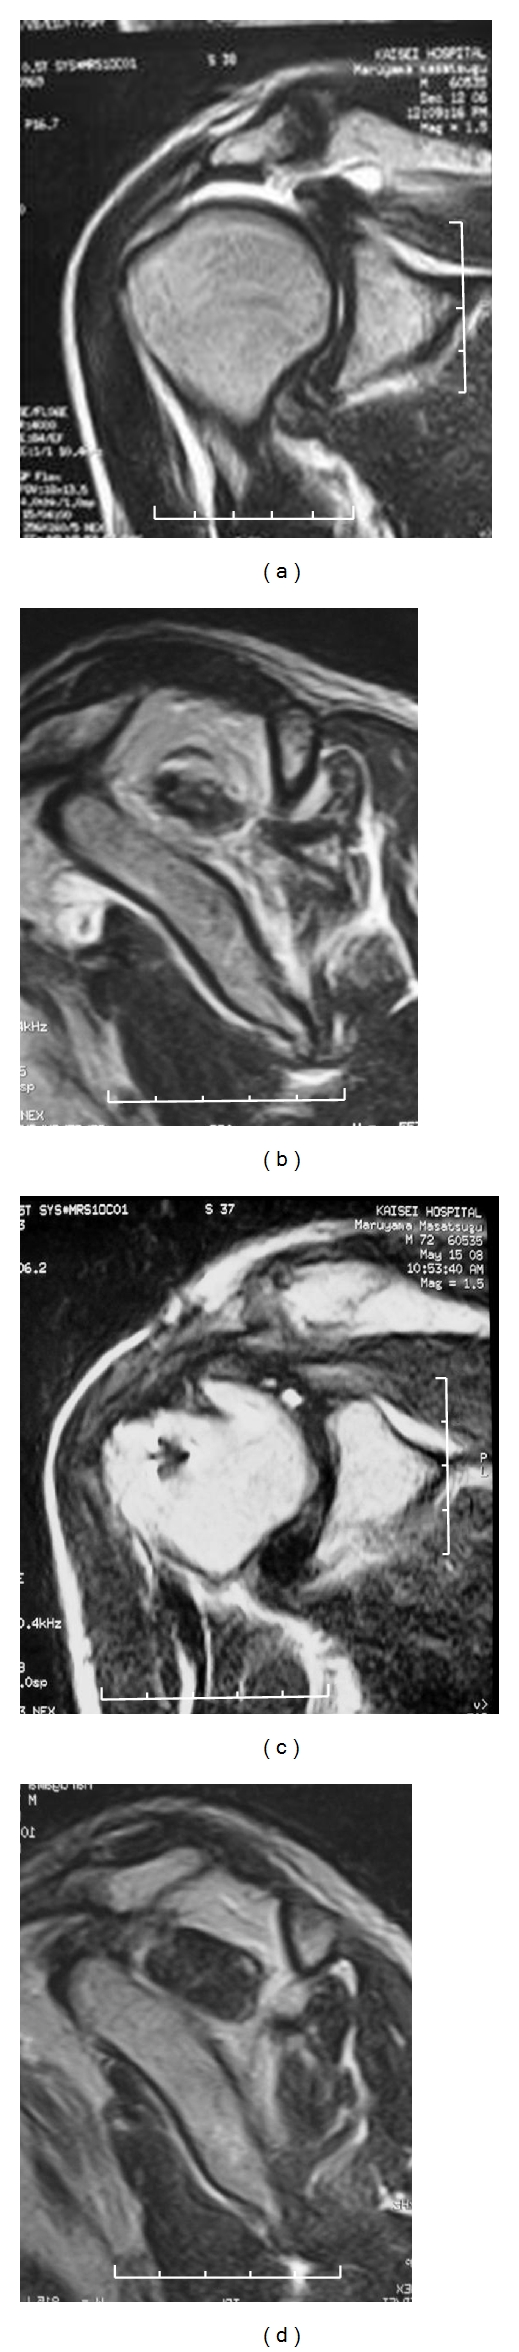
Case 2. (a) Pre-op MRI; coronal, (b) sagittal. (c) Post-op MRI (18 months after surgery); coronal, (d) sagittal.
